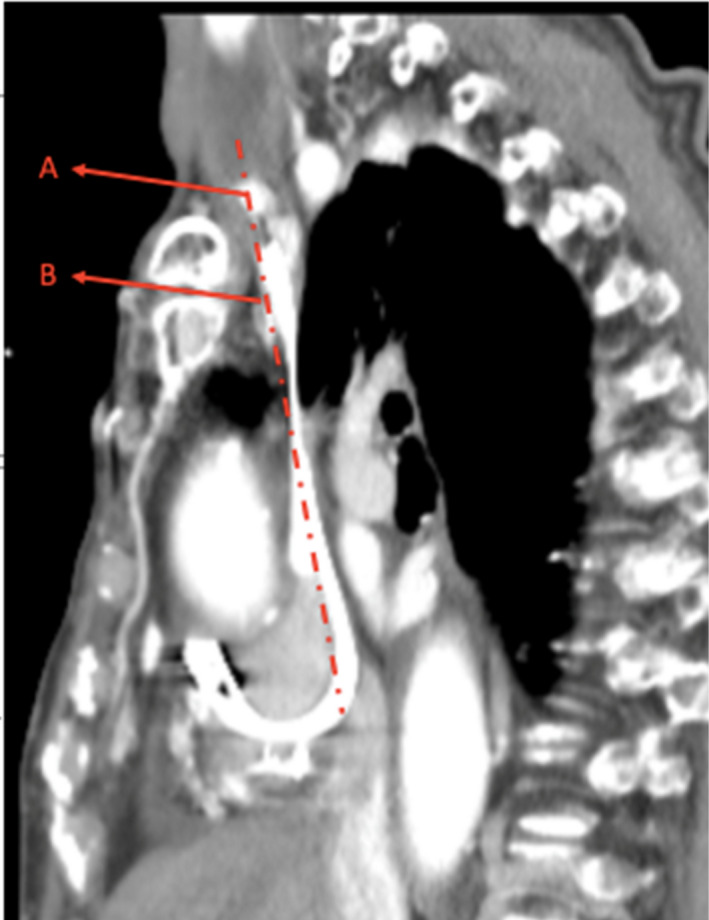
Sagittal plane view of CT Venogram of our patient in 10/2019 pre‐extraction. Vertical line marks VCS/BCV posterior to clavicula. A supraclavicular exit, B infraclavicular exit
